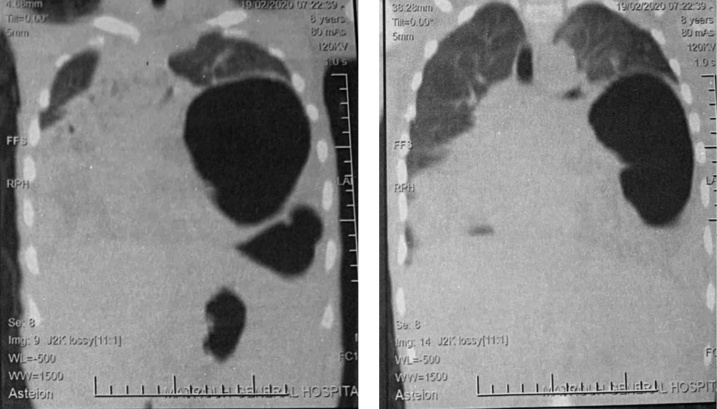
CT scan with coronal reconstruction showing distended stomach, due to organoaxial gastric volvulus.
